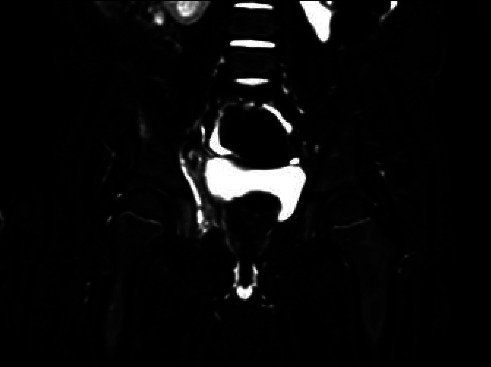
MRI pelvis. Right hemipelvis edema with probable osteomyelitis. Intraosseous abscess within the right ischium with surrounding myositis.
